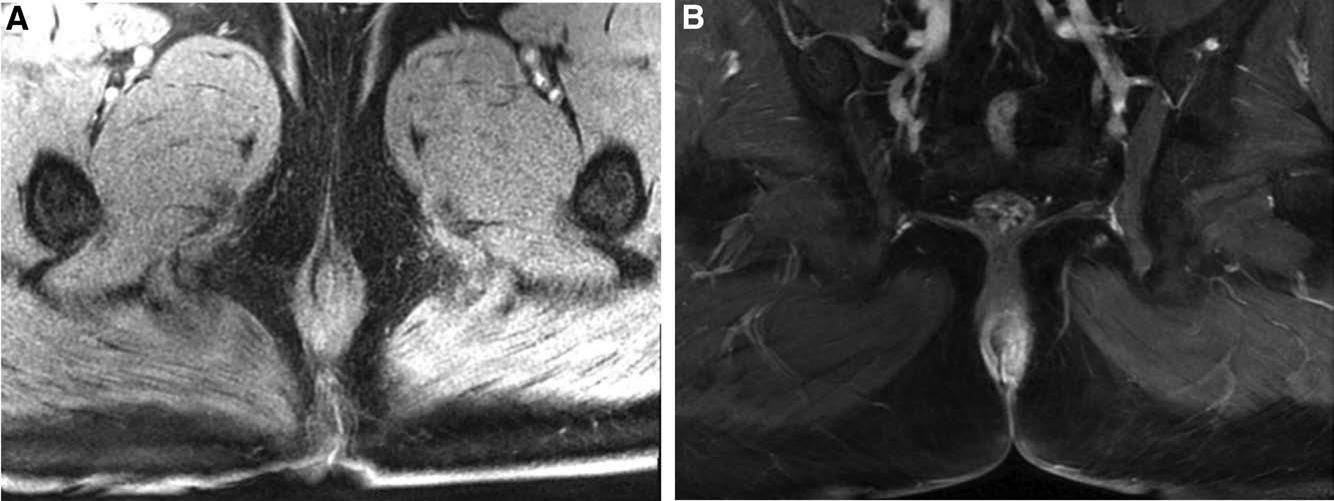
Preoperative MRI of the anal canal with plain scan, diffusion, and dynamic enhancement images: (A) an abnormal signal is observed at the 5 o’clock position (lithotomy position) within the internal anal sphincter, suggestive of the fistula’s internal opening. (B) Approximately 3.6 cm from the anal verge, 2 fistula tracks are seen descending through the intersphincteric branch at the 5 o’clock and 7 o’clock positions. Lithotomy position: the anal canal is conceptualized as the center of a clock face, with the 12 o’clock position corresponding to the patient’s ventral side (anterior) and the 6 o’clock position corresponding to the patient’s dorsal side (posterior). MRI = magnetic resonance imaging.
